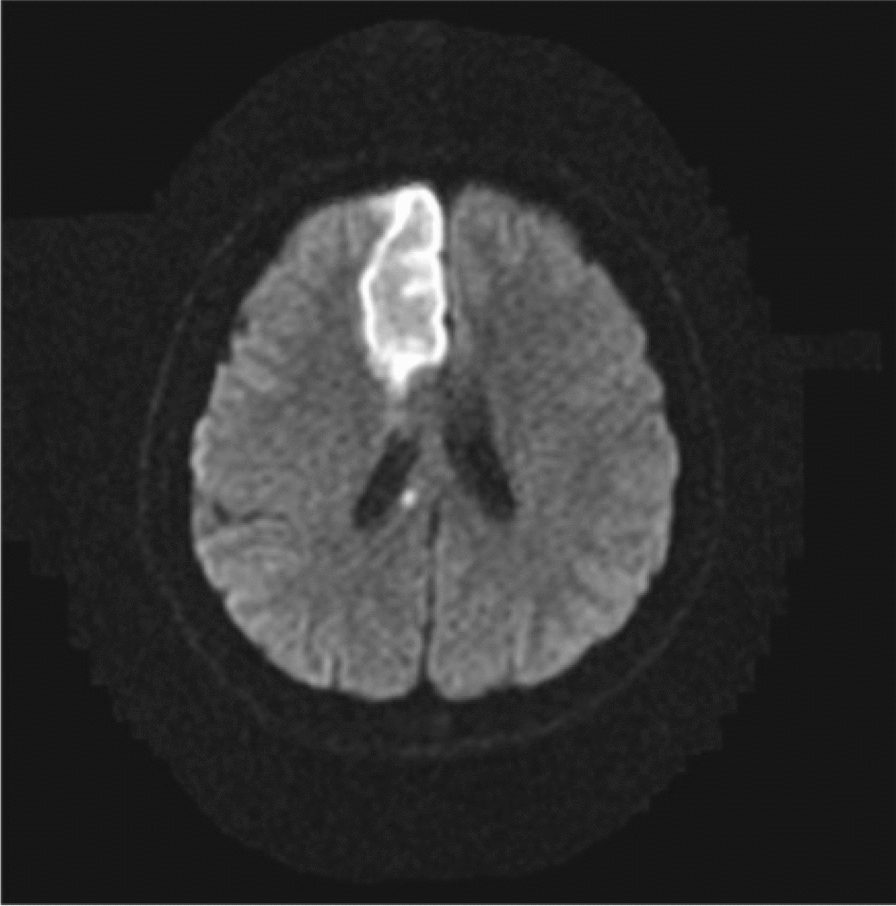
Diffusion-weighted magnetic resonance image showing acute infarction in the right frontal lobe & adjacent corpus callosum. [43] Copyright © 2012 by Korean Academy of Rehabilitation Medicine
